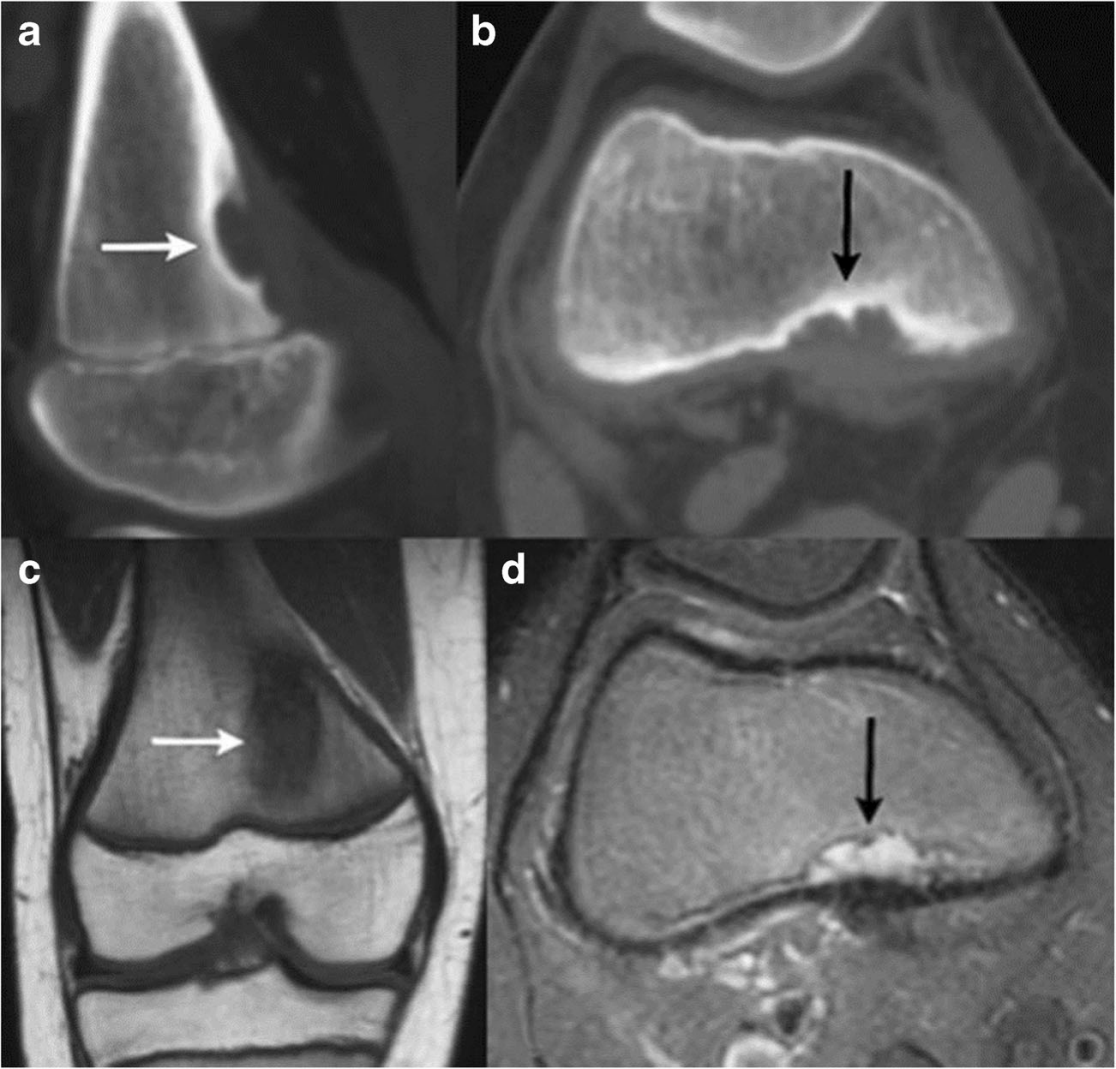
Cortical desmoid at the postero-medial aspect of the left femoral metaphysis in a 10-year-old girl. Sagittal a and axial b CT images show a scalloped radiolucent lesion with a thin sclerotic rim (*arrow*) along the posteromedial distal femoral metaphysis. Coronal T1 c and axial T2 fat-suppressed d images demonstrate low signal on T1 and high signal on T2 (*white arrows* on c and *black arrows* on d)On MR, these lesions usually have a low to intermediate signal on T1WI and PDWI, intermediate to slightly high signal on T2WI, and high signal on FS T2WI. A thin border of low signal on T1WI and T2WI is often seen at the inner margin of the lesion corresponding to a thin zone of sclerosis seen on plain films and/or CT. Bone marrow deep and peripheral to the lesion may have a slightly high signal on FS T2WI. The lesion itself and the surrounding marrow may enhance with gadolinium [2].
